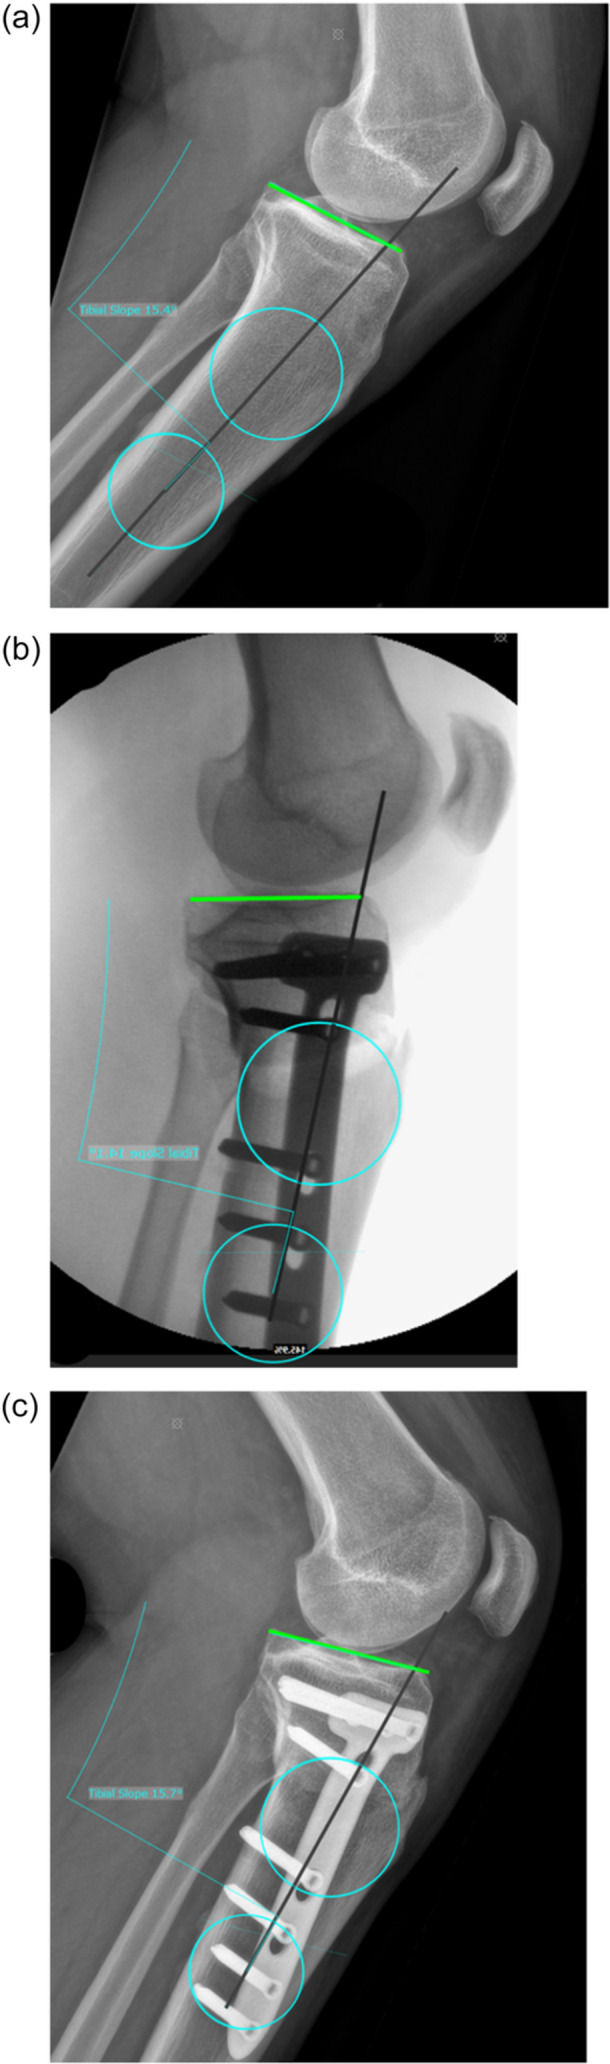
Preoperative, intraoperative and postoperative posterior tibial slope (PTS) measurements on short lateral knee radiographs. (a) preoperative short lateral knee radiograph, (b) intraoperative short lateral knee radiograph, (c) postoperative short lateral knee radiograph. To identify the proximal anatomical axis of the tibia, two circles were placed on the proximal tibial shaft with their centres at 5‐cm (proximal light blue circle) and 10‐cm (distal light blue circle) distal to the knee joint line. Each circle was drawn tangent to the anterior and posterior cortices of the proximal tibia and the line connecting their centres (black line) defined the proximal anatomical tibial axis. A line tangent to the articular surface of the medial tibial plateau (green line) was drawn. PTS was then defined as the angle between this plateau tangent and the proximal anatomical tibial axis.
